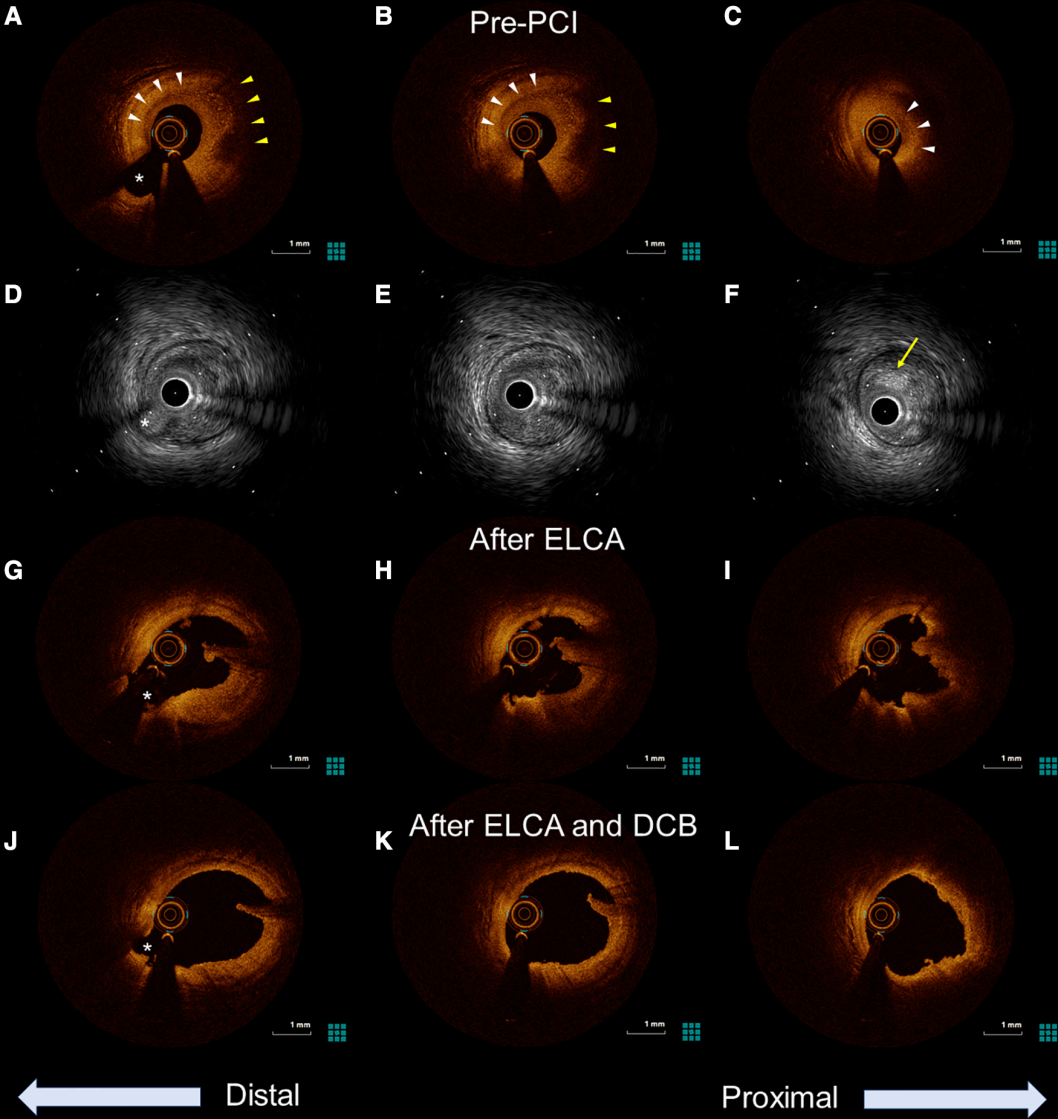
Optical coherence tomography (OCT) and intravascular ultrasound (IVUS) images of the culprit lesion from distal to proximal. The asterisk (*) marks the second diagonal branch as a landmark. (A–C) Pre-percutaneous coronary angiogram (PCI) OCT revealing the presence of healed plaque (white arrowhead). The partial accumulation of macrophages was also identified in the healed plaque (yellow arrowhead). Minimum lumen area was 0.87 mm^2^. (D–F) Pre-PCI IVUS image showing a high-intensity marginal irregular mass in the culprit site (yellow arrow), suggesting thrombus rather than lipid plaque or necrotic tissue. (G–I) OCT examination in the LAD after excimer laser coronary angioplasty showing significant thrombus reduction and a preserved lumen size. (J,K) Further lumen expansion was achieved with the addition of balloon dilatation, and the minimum lumen area expanded to 5.49 mm^2^.
